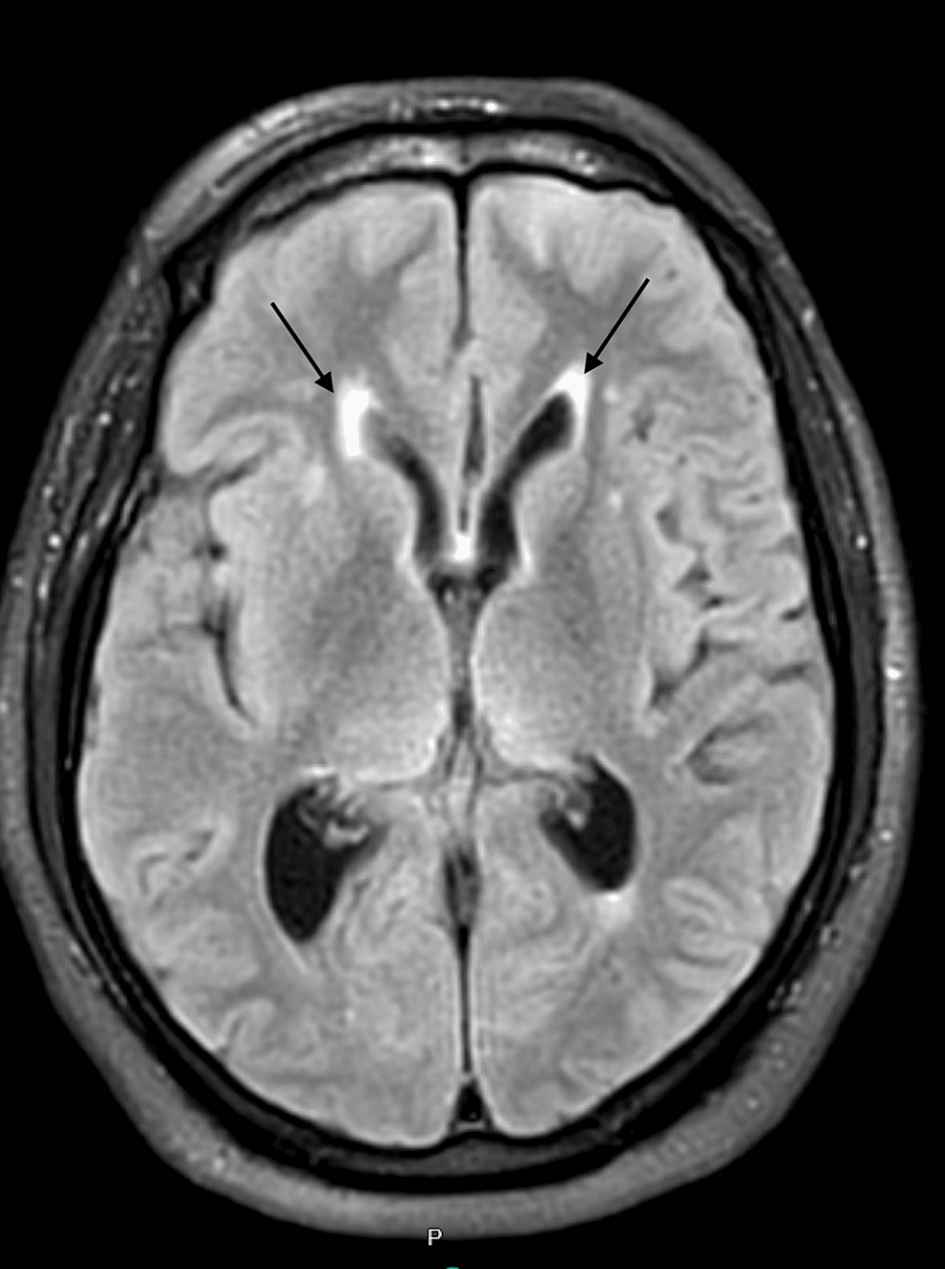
MRI of the brain showing abnormal fluid-attenuated inversion recovery (FLAIR) signals in the periventricular regions (black arrows)
